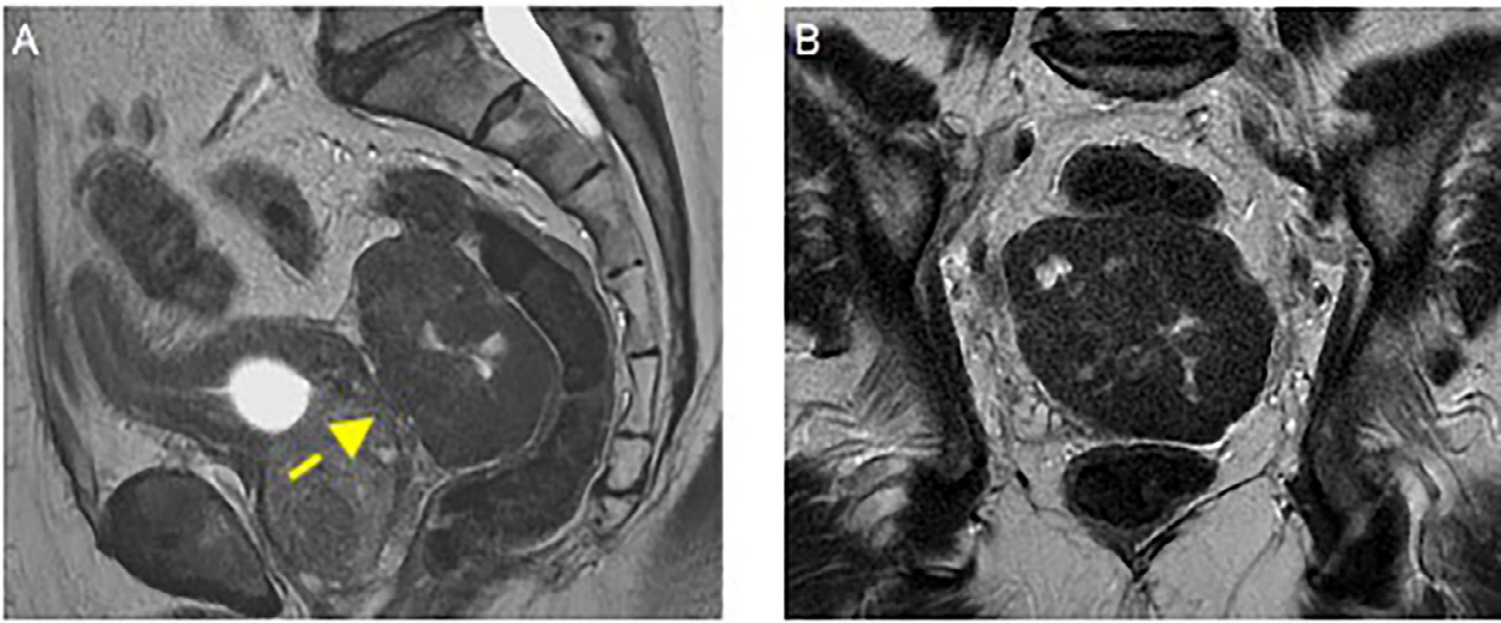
Sagittal (A, arrow) and coronal (B) Axial Pelvic MRI T2-w, reveal relationship with the main solid and hollow peritoneal organs, from which it does not originate.
